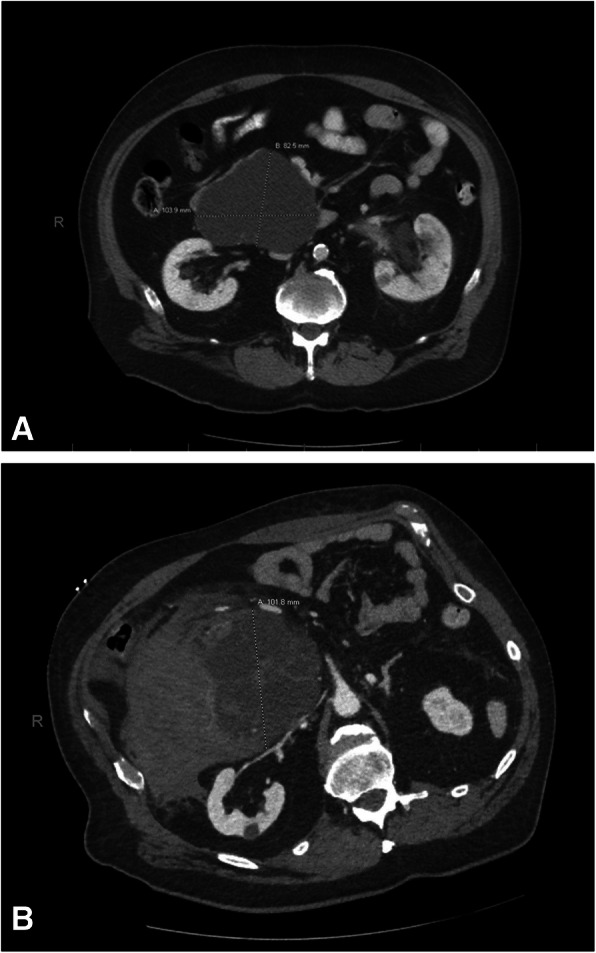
a Representative image of CT from 2015 demonstrating 10 × 8 cm septated cystic lesion in the head of pancreas. b Image of CT from 2020 demonstrating complex, septated cystic mass now measuring 14 × 6 cm, with focus of active hemorrhage
